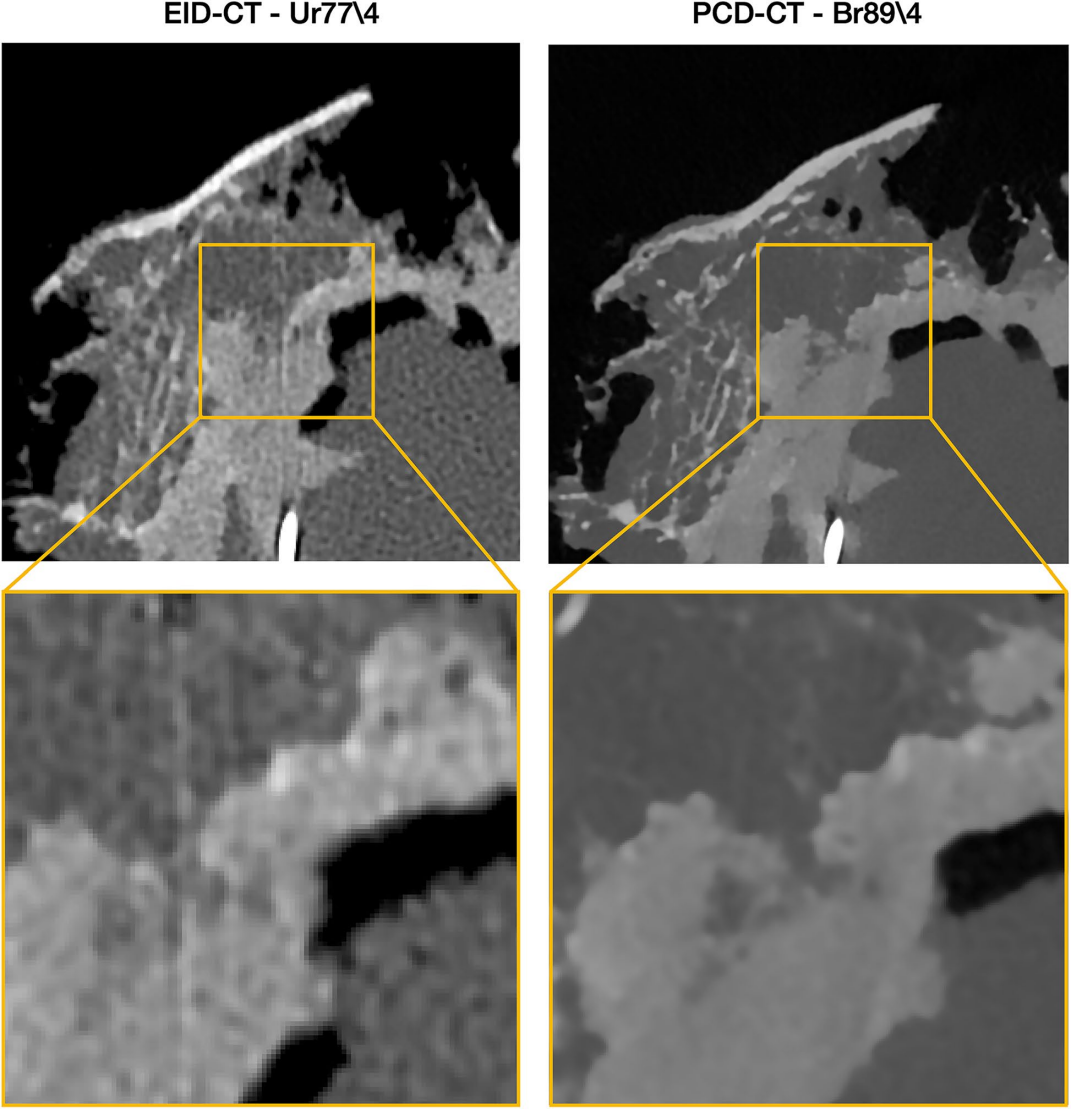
Example of images obtained with EID-CT using Ur77 reconstruction kernel with iterative strength (ADMIRE) 4 and 0.4 mm slice thickness (left) and photon-counting detector computed tomography (PCD-CT) using a Br89 kernel with quantum iterative strength (QIR) 4 and 0.2-mm collimation (right). Differences in visualization quality of the cement–bone interface in the cemented cup can be clearly observed
